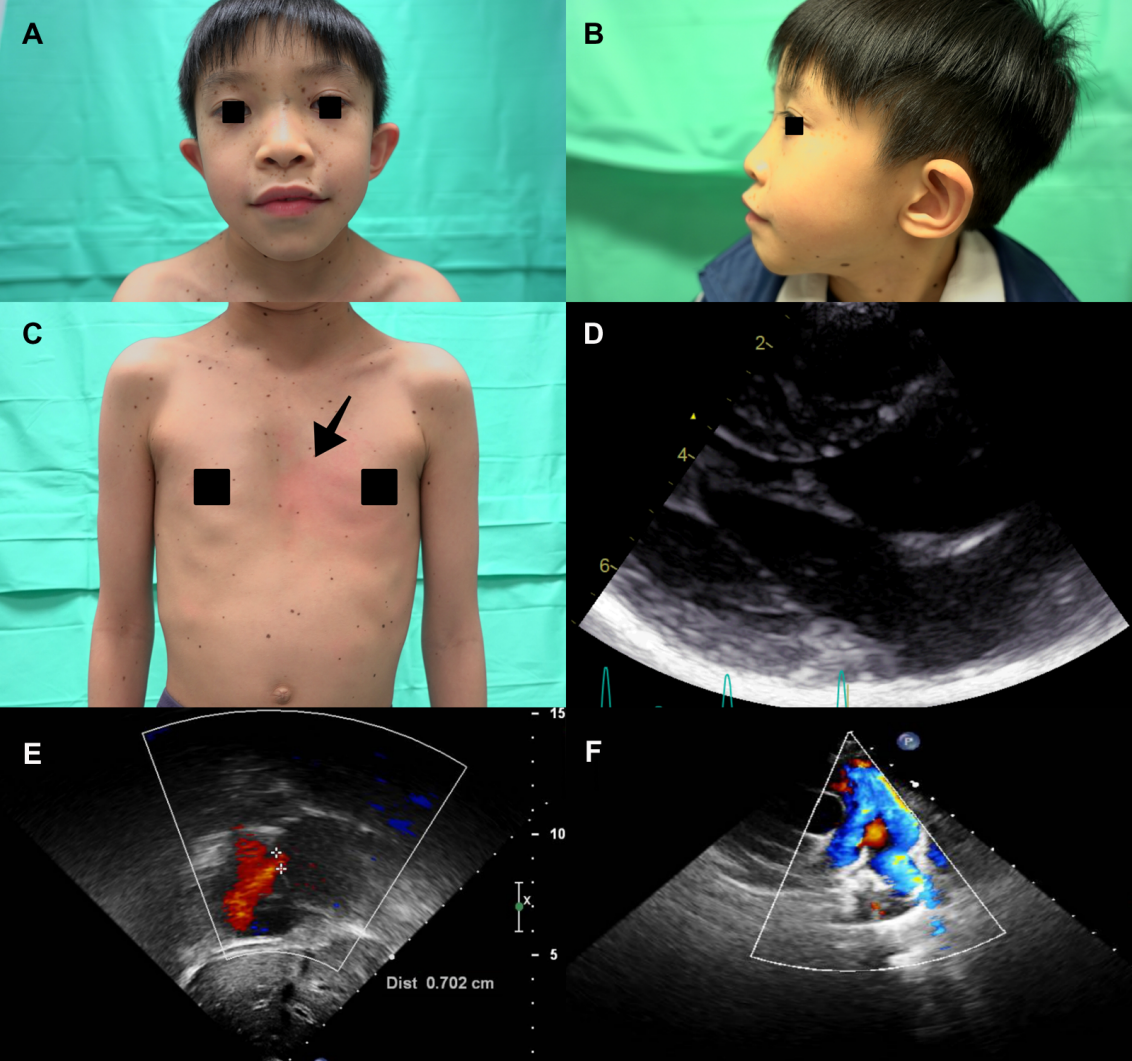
(A) The proband's son (individual III-2) presented with diffuse lentigines over the face, thick lips and ocular hypertelorism. (B) Low set ears and (C) Diffuse lentigines over the trunk and pectus excavatum (arrowhead) were also revealed. Echocardiography showed (D) septal wall hypertrophy without intra-cavity or left ventricle outflow tract obstruction. (E) Secundum atrial septal defect (cross-mark) (defect size about 7 mm, shunting from left to right). (F) Narrowing color-flow at the right pulmonary artery, indicating right pulmonary artery stenosis.
